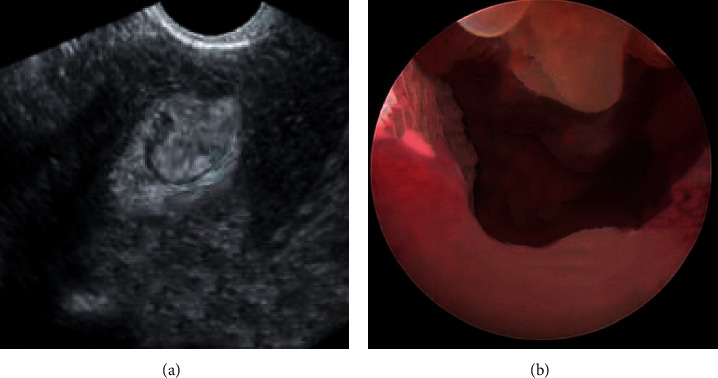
(a) Comparison of ultrasound; (b) hysteroscopy image in patients with endometrial polyps.
